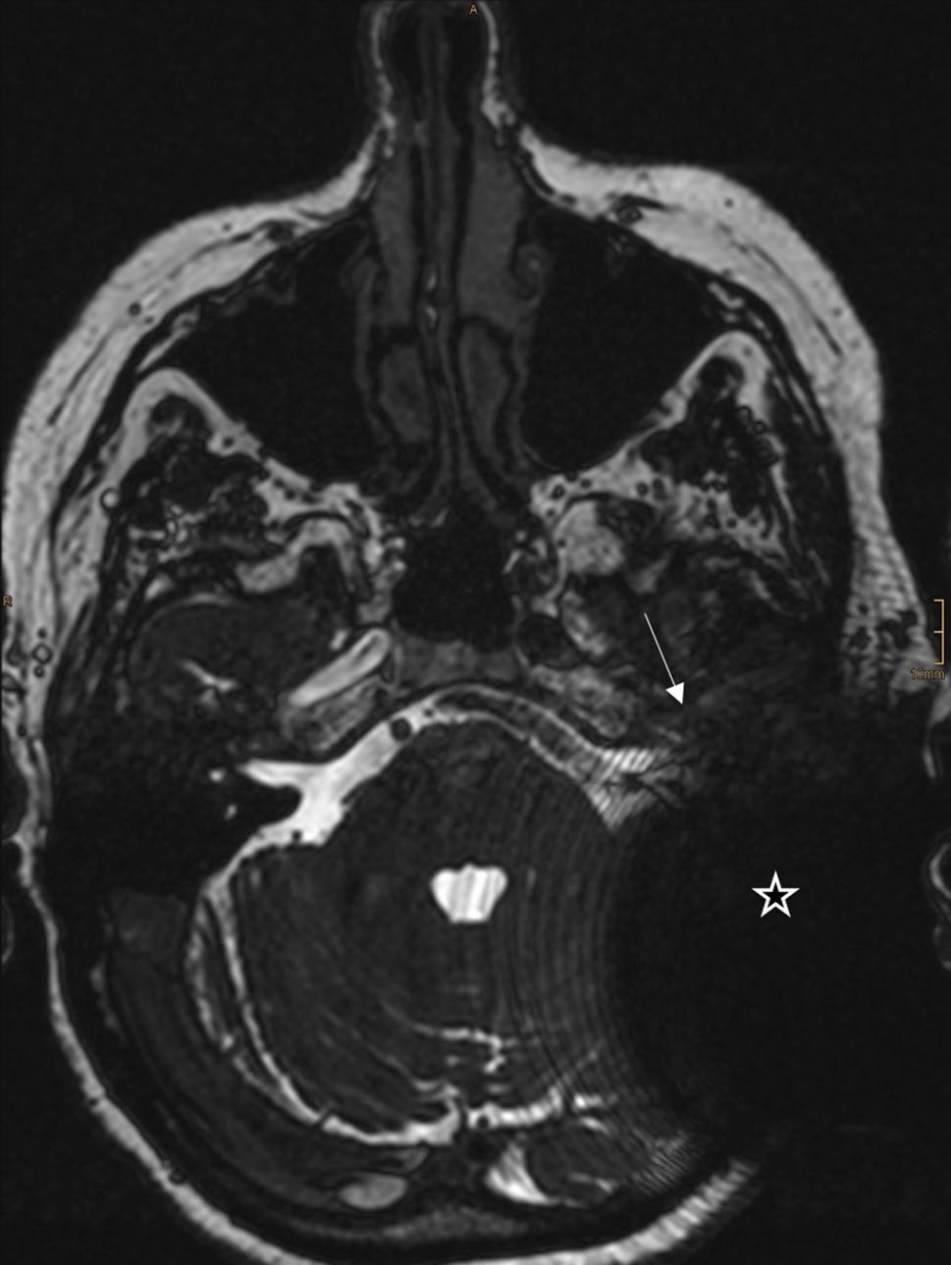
MRI CISS, axial, postoperative image. The arrow points at the cochlea (hardly visible), the star is placed in the large artifact created by the cochlea implant. This image was rated as Grade 0—poor image quality
